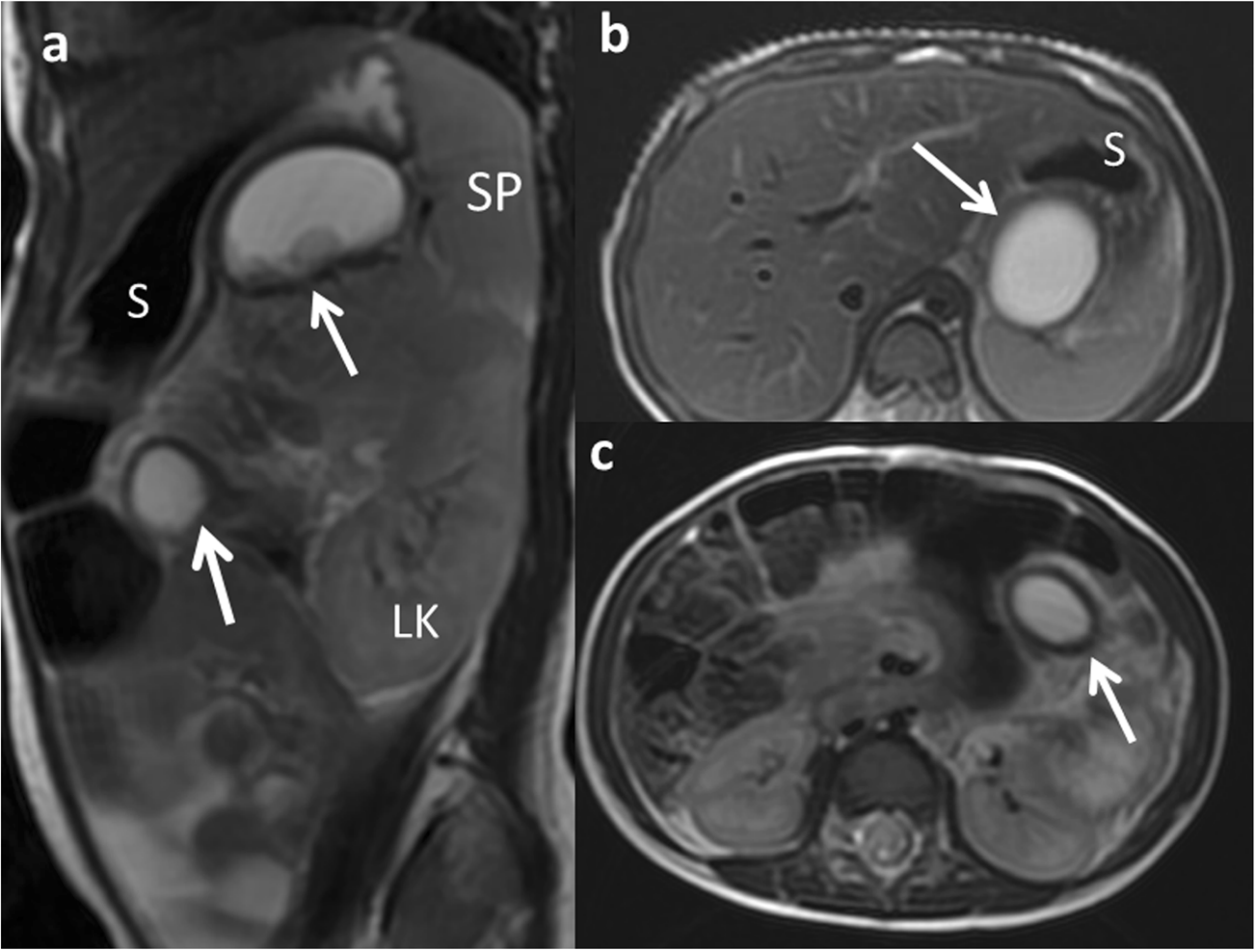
A 2-year-old girl with a splenic lesion (not shown) is studied with abdominal MR. a FSE T2 sagittal MRI: two similar cystic lesions are found (*arrows*). *S* stomach, *LK* left kidney, *SP* spleen. b, c FSE T2 axial MRI: a gastric and a jejunal duplication cyst are shown (*arrows*) in both images. Surgical findings: multiple EDCs
